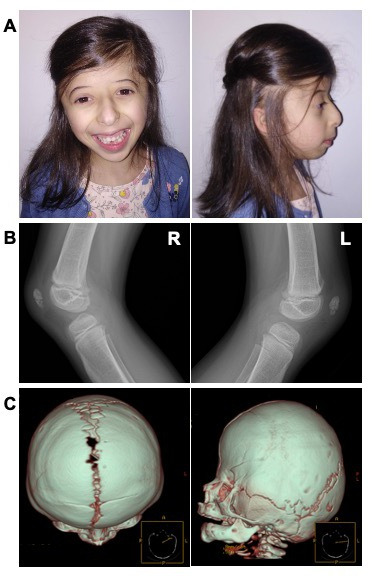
Clinical findings of an individual with a homozygous missense *GINS2* variant. (A) Craniofacial features of Meier-Gorlin syndrome at 6 years of age, including microtia, thin eyebrows, a narrow nose with a convex nasal ridge, microstomia, full lips and microretrognathia. (B) Lateral radiographic view of both knees at 7 years of age, showing hypoplastic patellae. (C) 3D reconstruction of cranial CT scans at 5 months old, demonstrating an incomplete premature fusion of coronal sutures. L, left knee; R, right knee.
